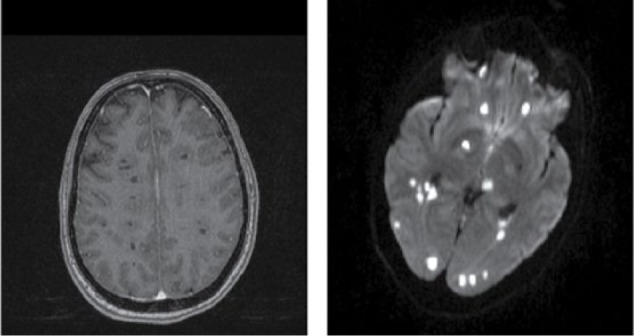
MRI images from day of admission. Post contrast T1 weighted image (left); diffusion weighted image (right).
